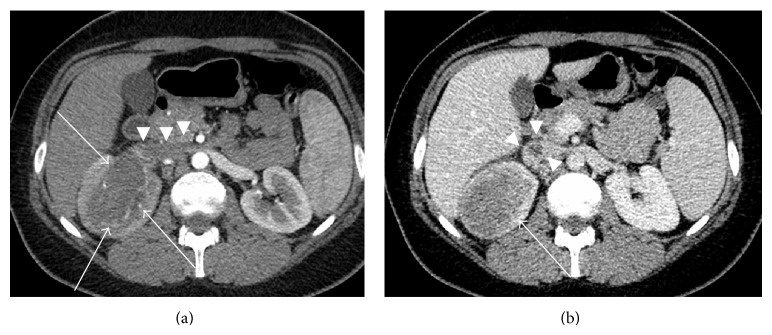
(a, b) Axial venous phase postcontrast CT images (a, b) from the mid-portion of the kidneys. There is a hypoattenuating mass lesion involving the right central part of the kidney with sinus extension (arrows). Hypodense thrombus materials are seen in both right renal vein and inferior vena cava (arrowheads).
